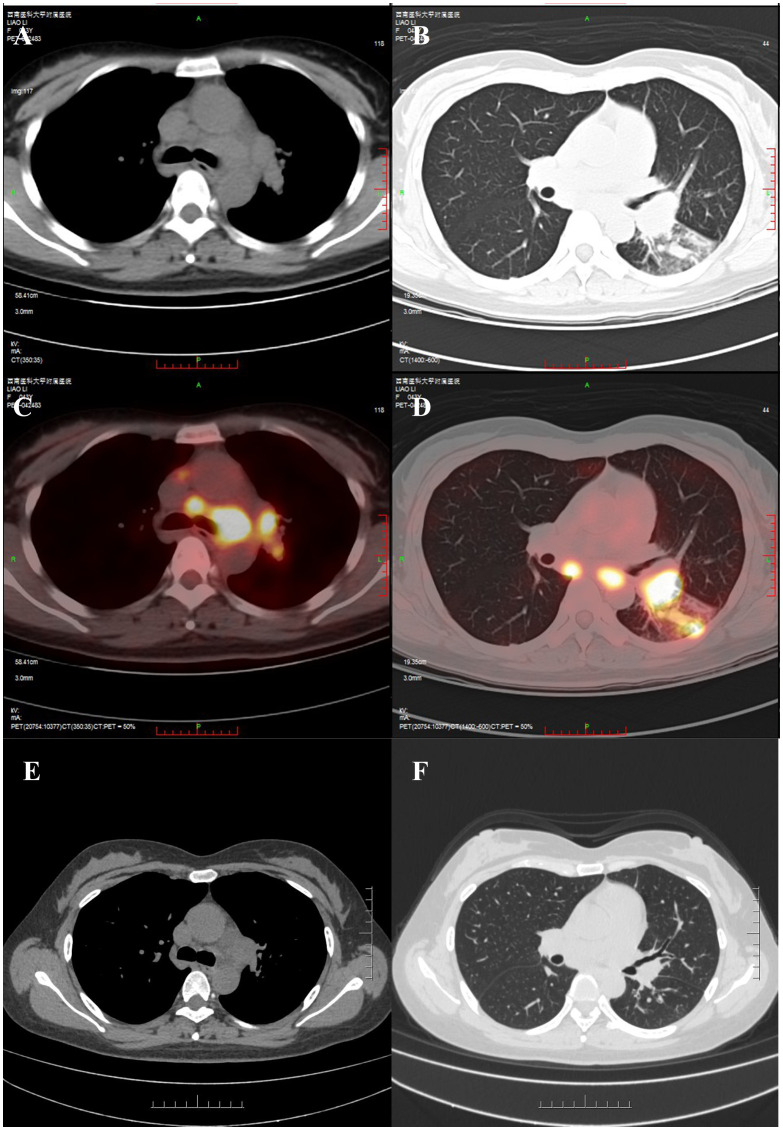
Initial imaging revealed a left lower hilar mass with increased glucose metabolism (measuring approximately 3.5 cm × 2.5 cm in maximum cross-section), along with enlarged and hypermetabolic mediastinal and hilar lymph nodes. A solid nodule in the dorsal segment of the left lower lobe also exhibited hypermetabolism. No distant metastases were identified. (A, B) Chest CT; (C, D) PET-CT. (E, F) Follow-up imaging on January 4, 2024, showed significant regression of the lesions in the left hilum and the dorsal segment of the left lower lobe, as well as a decrease in size of some mediastinal lymph nodes.
